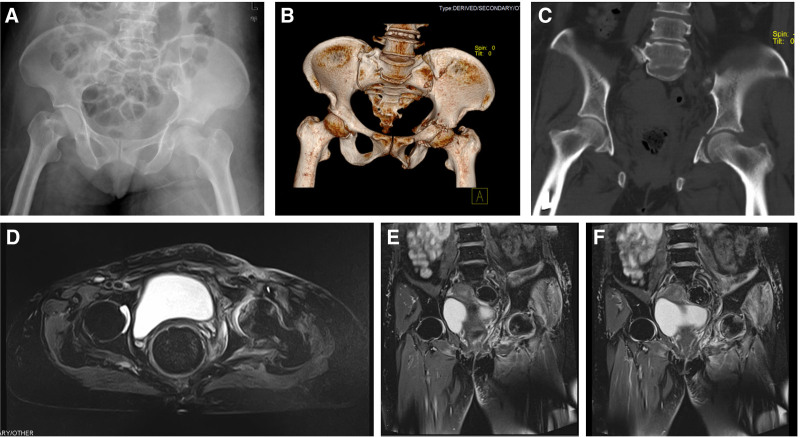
(A–C) The X-ray and computed tomography images showed that the left femoral head was in place, and the fracture site was not significantly displaced, with local bone callus growth. (D–F) The magnetic resonance imaging showed abnormal signals in the left femoral head, and the high signals were mainly located at the edge of the femoral head, considering the contusion.
